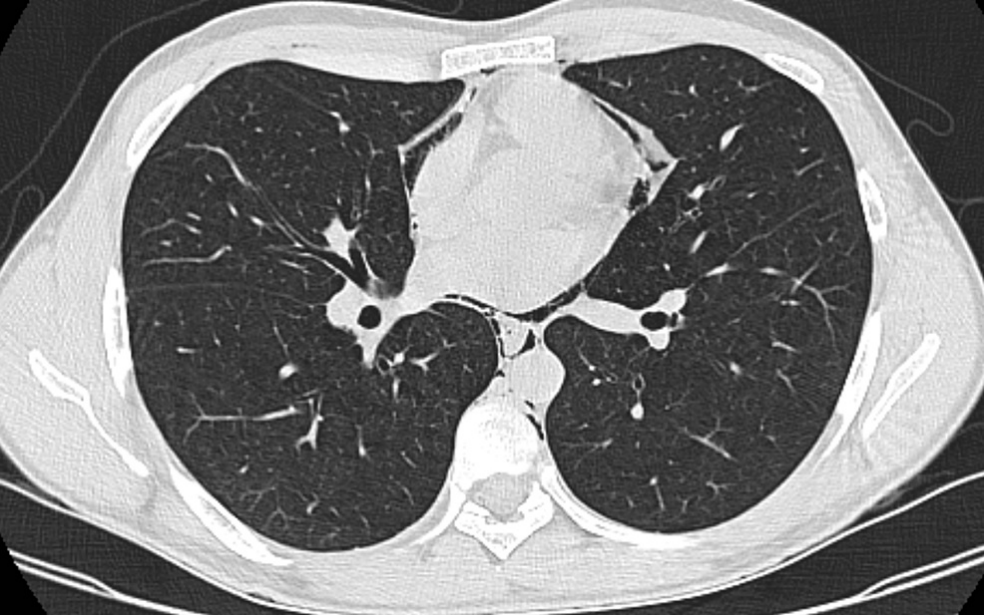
Computerized tomography (CT) scan The chest CT demonstrates the air outlining the inner surface of the mediastinal pleura and the mediastinal structures.
